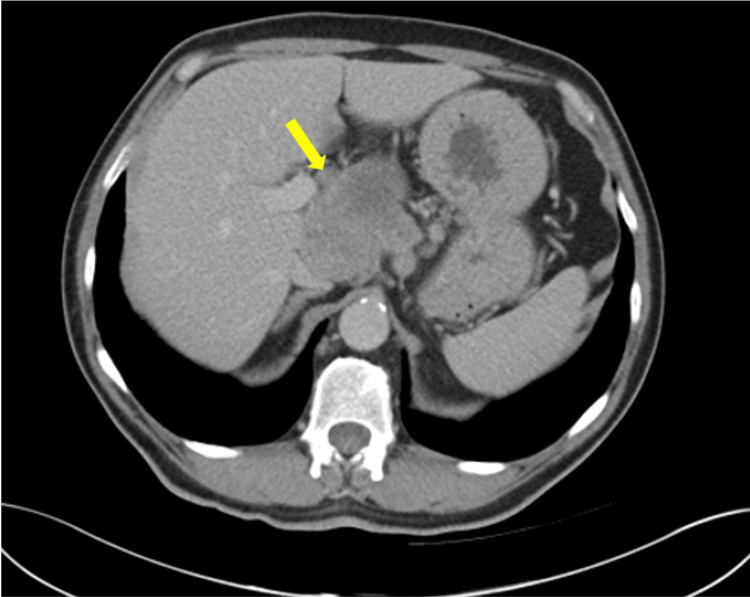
Contrast-enhanced axial CT at diagnosis showing a pancreatic mass centred in the neck of the pancreas (yellow arrow).
